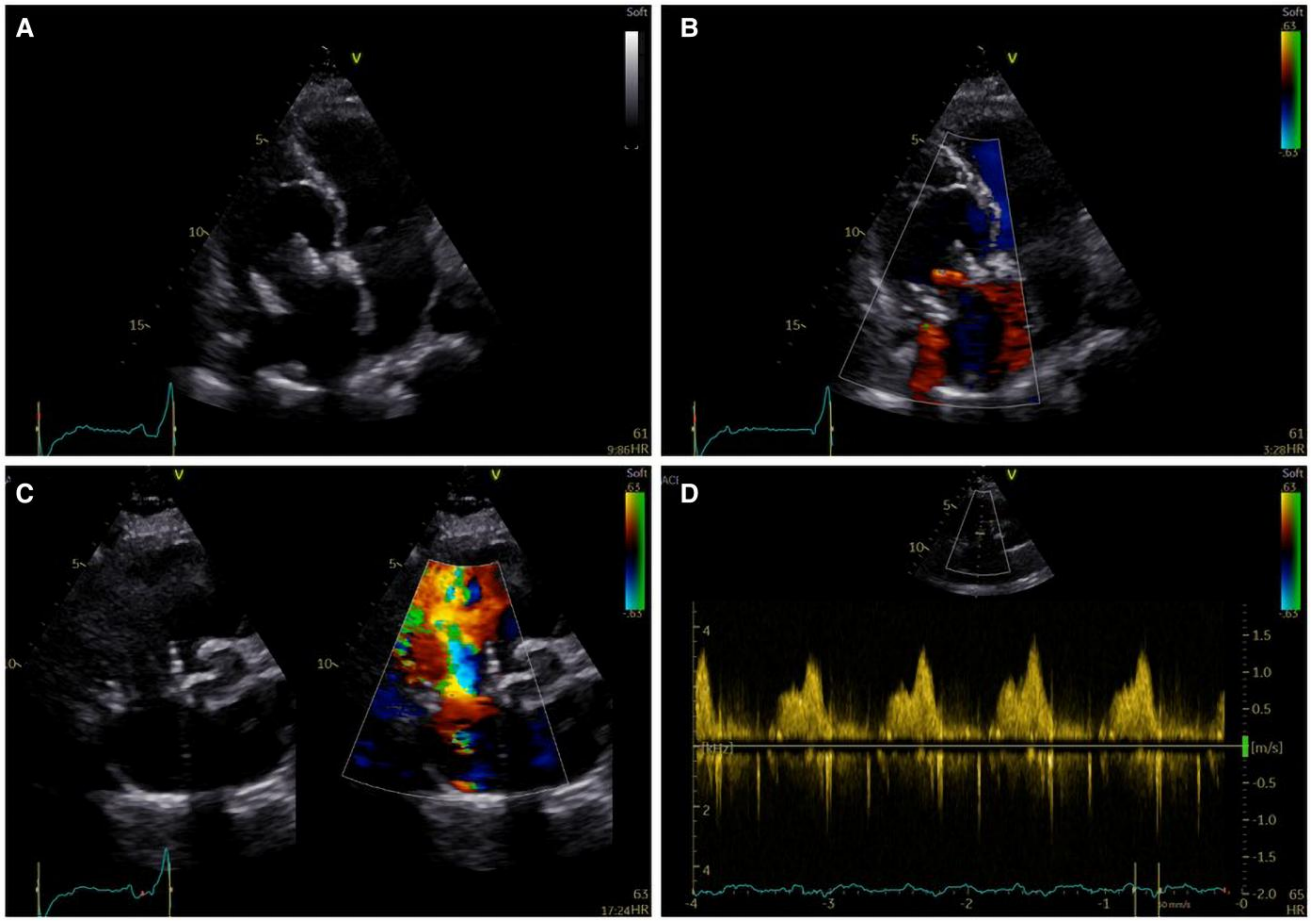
Apical four-chamber view by 2D echocardiography (*A*) and Doppler imaging (*B*) with a focus on RV inflow showing good function of the transcatheter valve in the tricuspid position. Short axis view by 2D echocardiography and Doppler imaging (*C*) with a focus on RV inflow showing laminar flow in the tricuspid valve prosthesis with no para-valvular or transvalvular regurgitation. (*D*) Continuous wave Doppler imaging showing good function of transcatheter valve in the tricuspid position with a mean gradient ∼1 mmHg at 65 b.p.m. RV, right ventricle.
